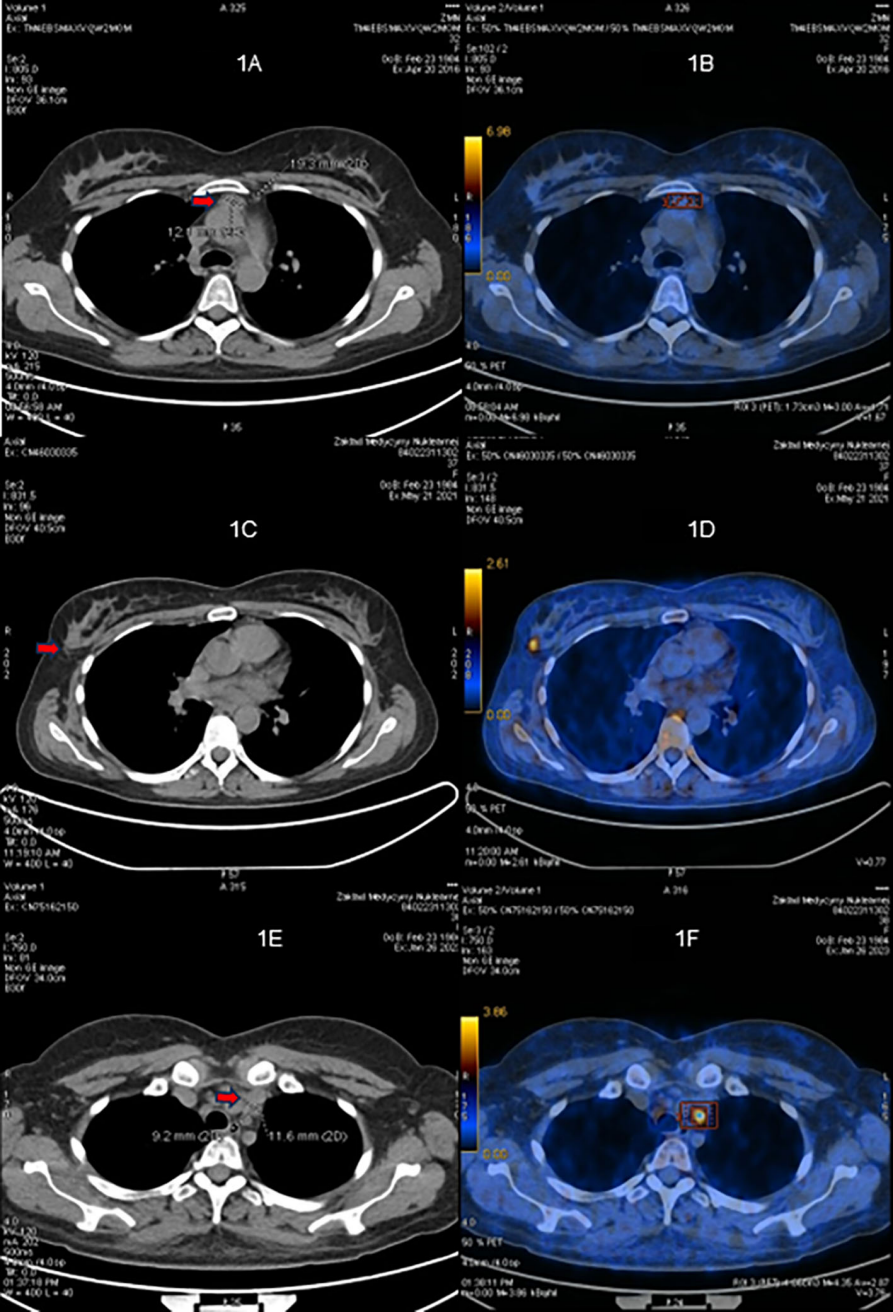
^68^Ga-DOTATATE PET/CT scans. (A, B) Before the first surgery (April 2016). (C, D) Before the second surgery (May 2021). (E, F) Before the third surgery (January 2023).
